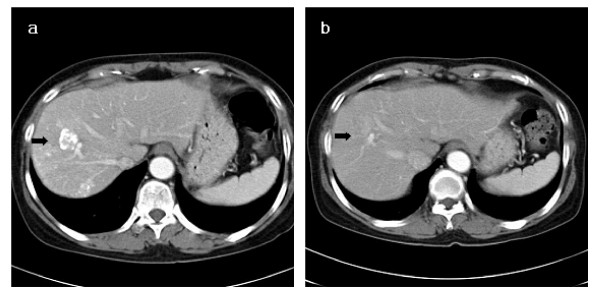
(a) The initial abdominal CT scan with the primary HCC indicated by the arrow. (b) CT scan seven months after SBRT. This patient was classified as PR at 5 months after SBRT. (SBRT, stereotactic body radiation therapy).
